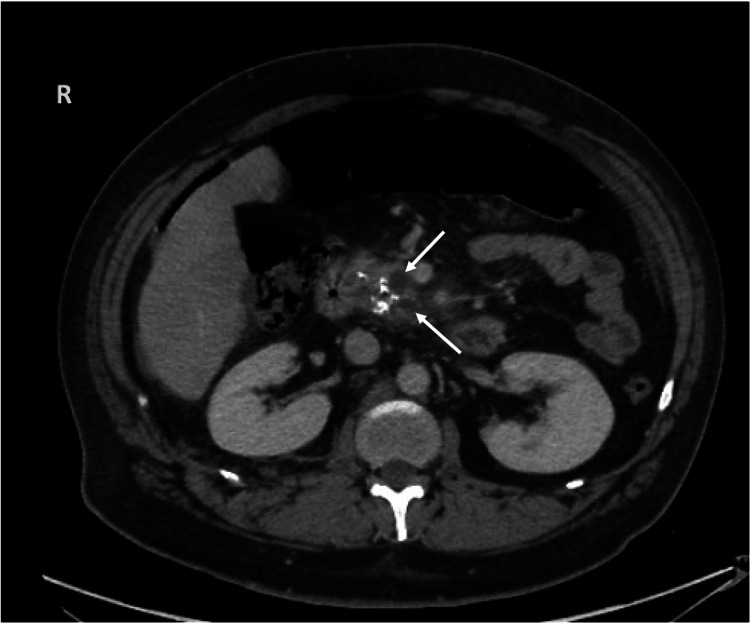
CT of the abdomen and pelvis with acute-on-chronic pancreatitis likely necrotizing with two cystic lesions/collections (arrows) within the pancreatic head and uncinate process. CT: computed tomography
